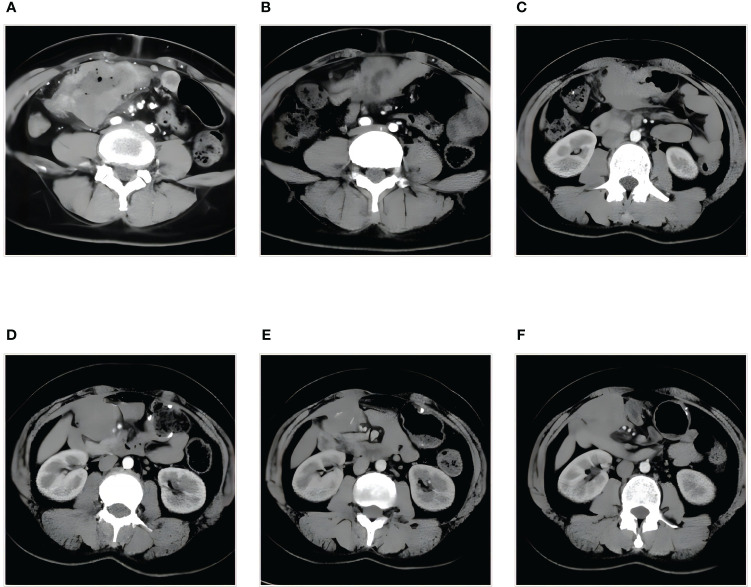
Treatment assessment by abdominal enhanced CT (A-F). (A) Diagnosis, the tumor infiltration depth is classified as T4. (B) PR, after two cycles of XELOX therapy. (C) SD, after four cycles of XELOX therapy. (D) PD, abdominal metastasis. (E) SD-, after four cycles of combination therapy. (F) SD+, after seven cycles of combination therapy. PR, partial response; PD, progressive disease; SD, stable disease.
